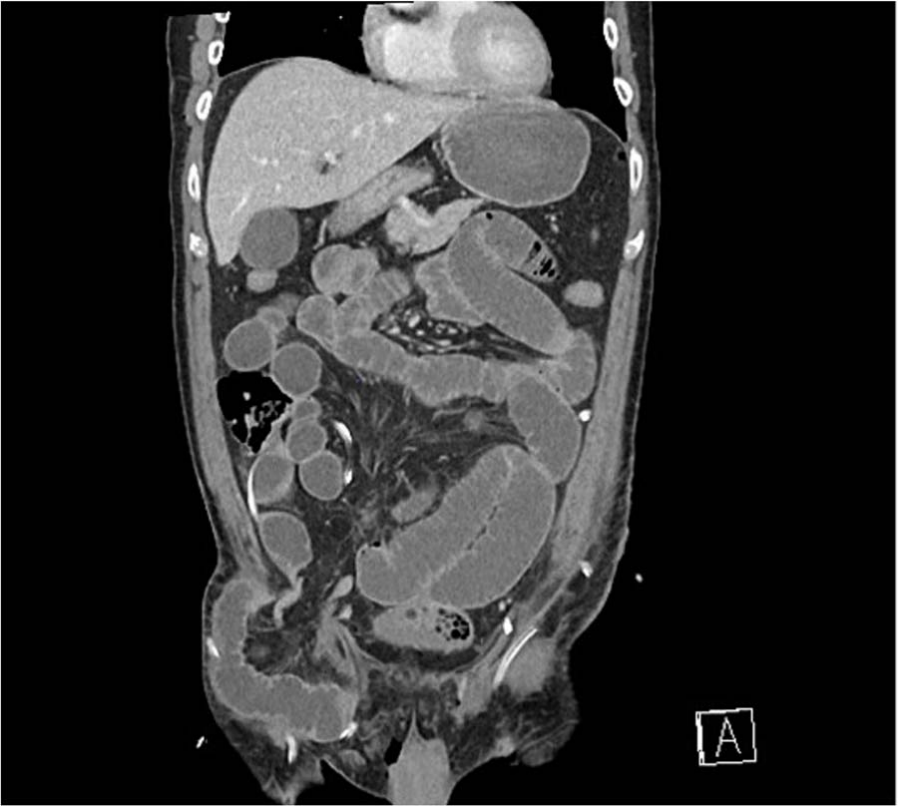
Coronal CT scan demonstrating small bowel herniating below the inguinal ligament, lateral to femoral vessels.
